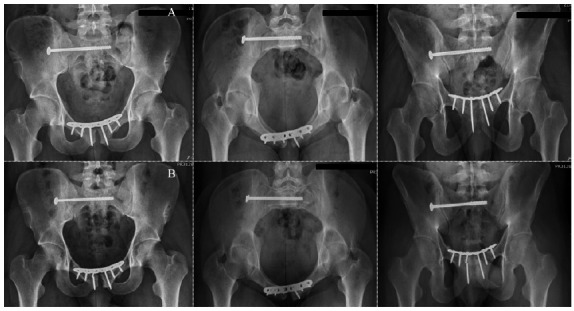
A. Postoperative radiographic views in AP, inlet, and outlet of the pelvis of a 22-year-old male patient who suffered type II APC injury after a motorcycle accident. The patient was operated on, and the pubic symphysis was fixed with a 4.5mm locked reconstruction plate. The sacroiliac joint injury was treated with a 7.0mm cannulated screw and washer in S1. B. AP, inlet, and outlet radiographs of the same patient taken at the last outpatient follow-up assessment showing adequate healing of the pelvic lesion despite a broken screw on the right side of the pubic symphysis.
